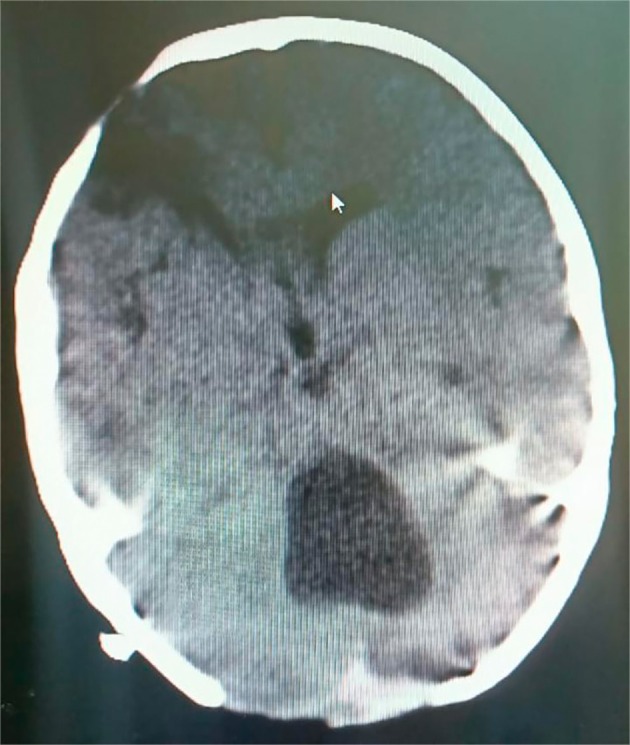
Postoperative CT scan showing craniotomy defects in the right occipital and right frontal bones, dilation of the ventricular chain with pneumocephalus, with VP shunt in place. The dilated fourth ventricle significantly reduced in size as the CSF flow became normal after fenestration.
